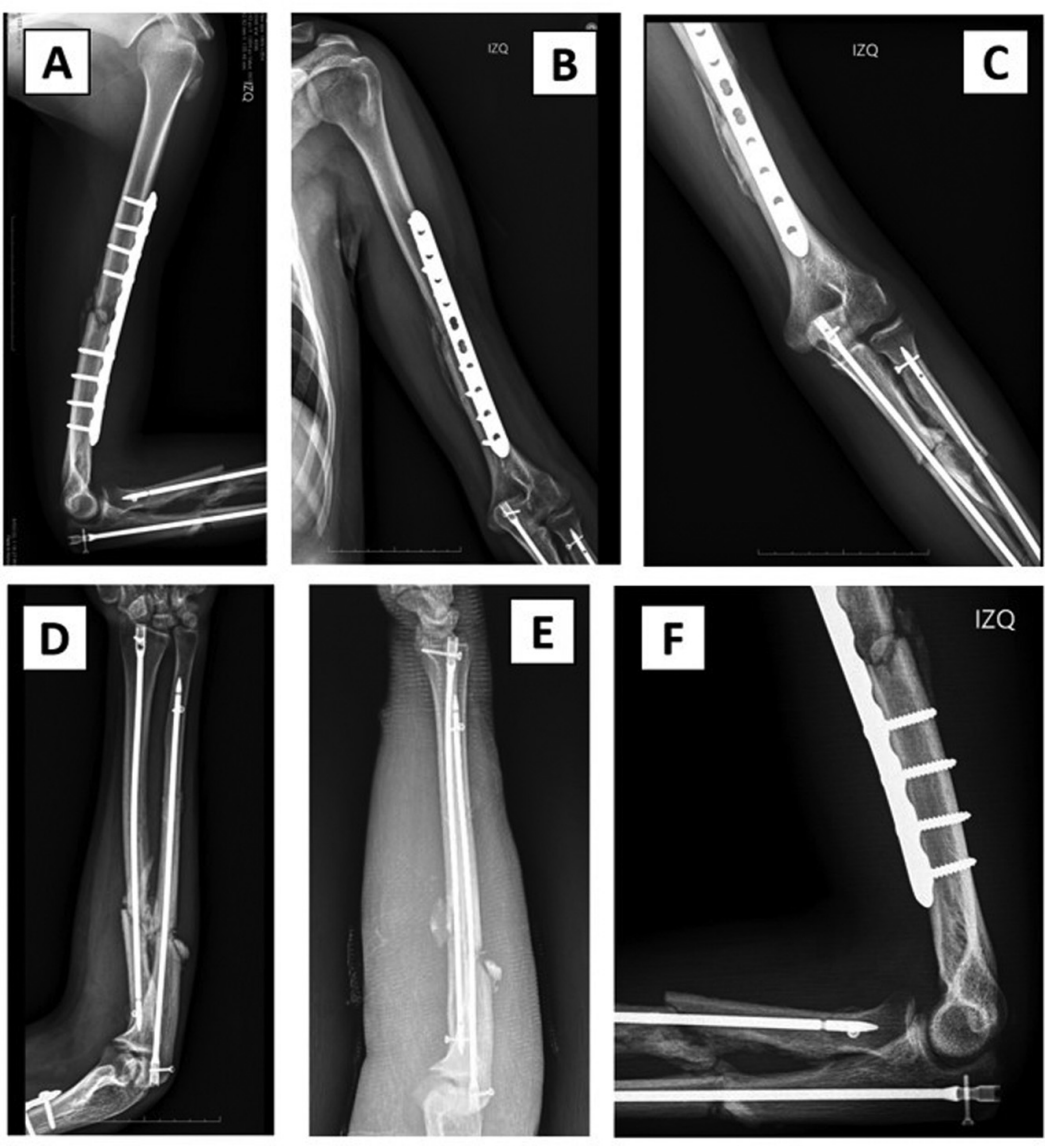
Postoperative radiographs. The fixation in the humerus with the LC-DCP plate is observed in the lateral (A) and anteroposterior (B) planes. X-rays of the elbow showing adequate restoration of the relationship between the humerus and forearm fractures in the anteroposterior (C) and lateral (F) planes. Stabilization of radius and ulna fractures with intramedullary nails in the anteroposterior (F) and lateral (E) planes.
